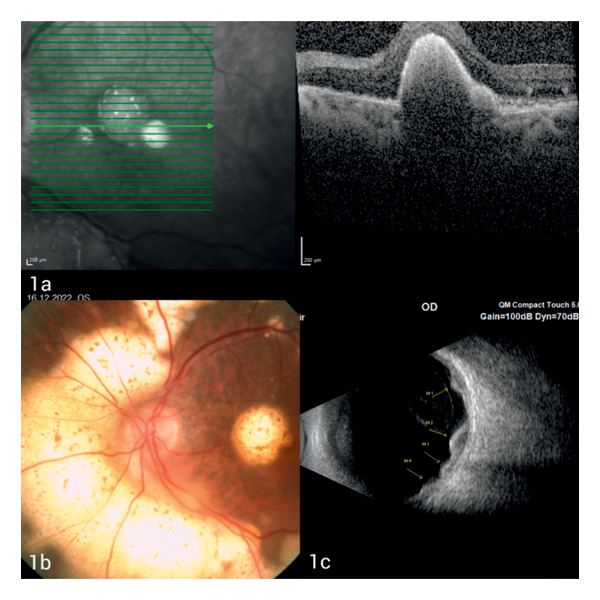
(a) Macular OCT of the left eye demonstrated large elevated subretinal infiltrates. (b) Dilated fundus examination revealed diffuse yellowish subretinal infiltrates around the optic disc and macular region. (c) Ophthalmic ultrasound showed subretinal mass lesions diffusely distributed in a scattered pattern.
